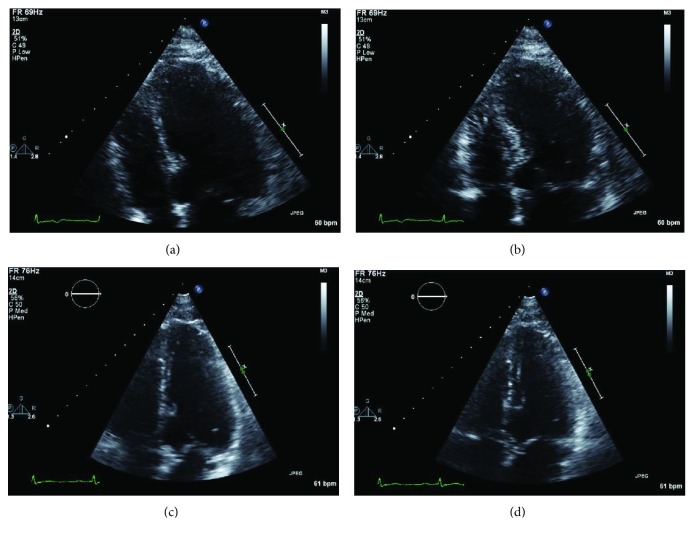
Transthoracic echocardiogram on admission and 6-week follow-up. Apical 4 chamber window at end-diastole (a) and at end-systole (b) showing apical dilatation during acute presentation. (c) Apical 4 chamber window at end-diastole at 6-week follow-up. (d) Apical 4 chamber window at end-systole at 6-week follow-up revealing resolution of apical ballooning.
